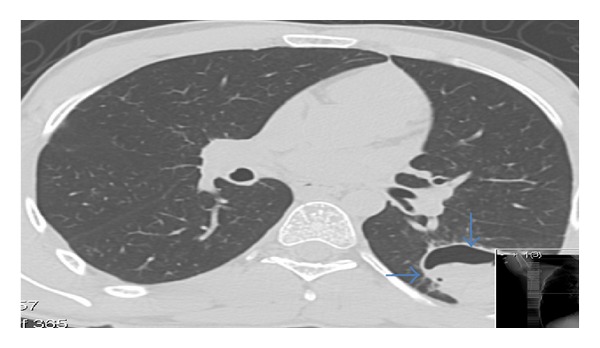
Chest CT: cavitation measuring 5.6 cm in the superior segment of the left lower lobe that contained soft tissue density (blue arrows).
